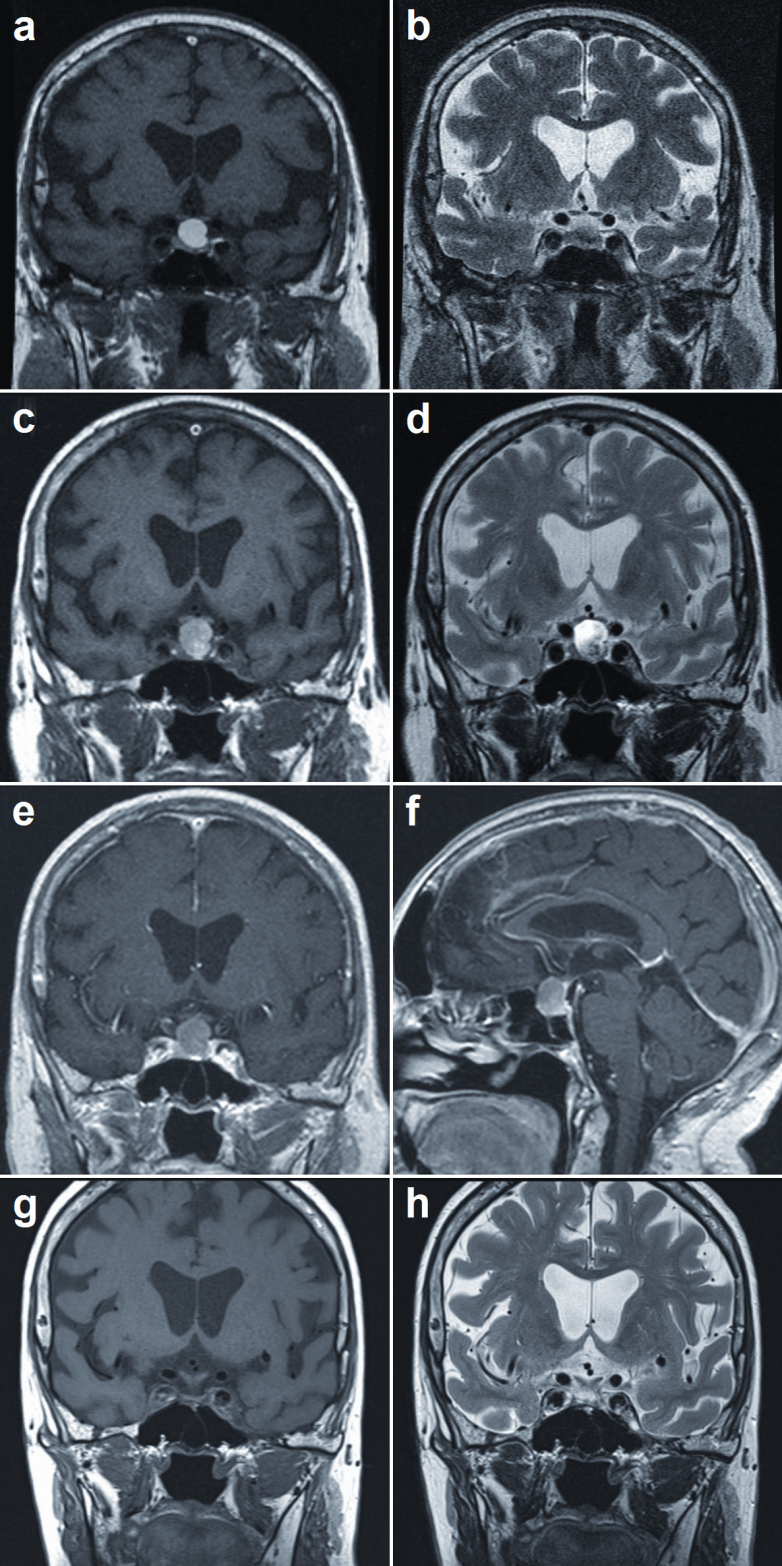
MRI images of patient's brain. (A, B) T1- and T2- weighted MRI scans taken two years prior to current presentation. (C) T1-weighted MRI scan of patient's brain, revealing a partly vesicular hyperintense, and slightly increased (compared to A and B) intrasellar and suprasellar mass of 16 mm in diameter, with progressive compression of the prechiasmatic portions of her optic nerves bilaterally. (D) T2-weighted MRI scan showing the vesicular portion as hypointense; normal pituitary tissue could not be clearly delineated. (E, F) There was no evident enhancement on T1-weighted imaging after intravenous administration of gadolinium. (G, H) An MRI scan taken 16 months postoperatively showed regular display of the remaining pituitary gland with good chiasmatic decompression and no signs of tumor recurrence.
